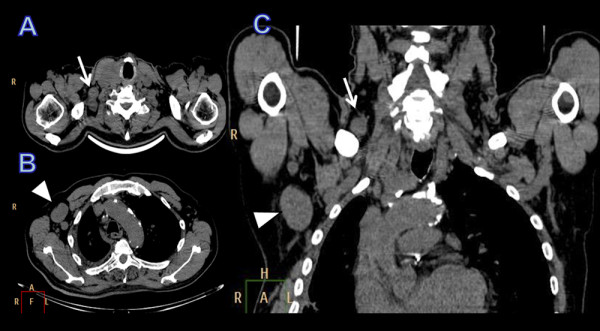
CT imaging in axial (a, b) and coronal (c) plane: Lymph node metastasis in the right axilla (arrowhead) and in the supraclavicular fossa (arrow).
